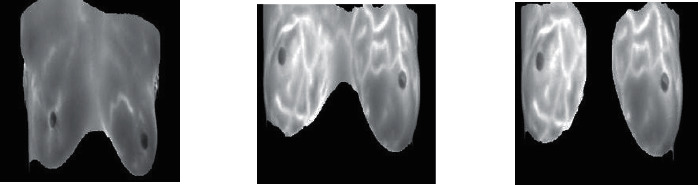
Preprocessed (227*∗*227) breast MRI scans.
